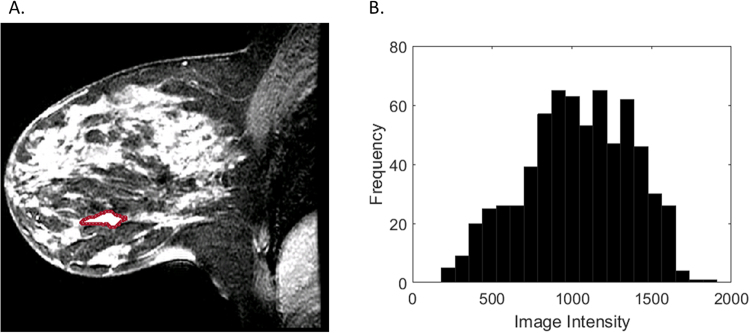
Representative HER2+ breast cancer requiring one re-excision on T1-weighted fat-suppressed first post-contrast images (A): solidity = 0.87, extent = 0.66. Histogram plot (B) of the intensity values from the region of interest highlighted in (A).
